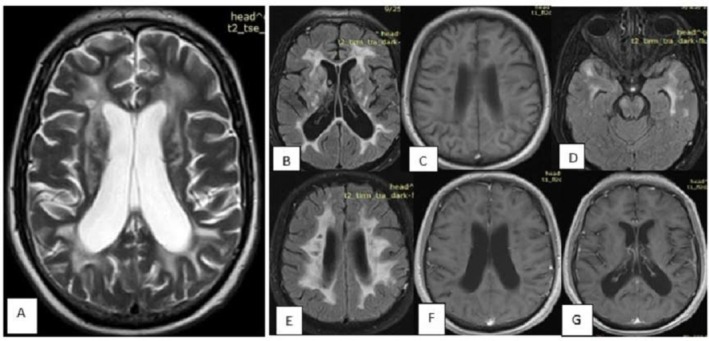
Initial brain MRI of the patient at age 23. (A) T2‐weighted image showing confluent hyperintensities in the periventricular and subcortical white matter. (B–D) FLAIR sequences demonstrating confluent hyperintensities in the periventricular, subcortical, anterior temporal, and external capsule regions, consistent with early diffuse leukoencephalopathy. (E) T1‐weighted image without contrast showing corresponding hypointensities. (F) Post‐contrast T1‐weighted image showing no abnormal enhancement.
